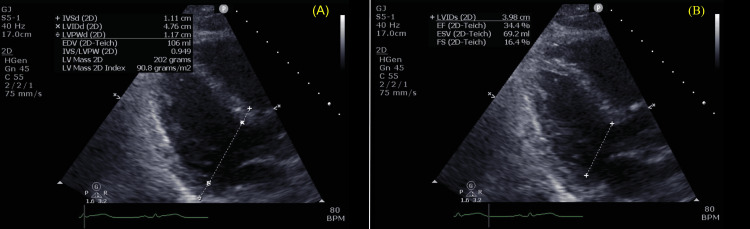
Transthoracic echocardiogram demonstrating systolic dysfunction (A) Modified four-chamber view at end-diastole. (B) Four-chamber view at end-systole. Teichholz-derived measurements demonstrate an impaired left ventricular ejection fraction (LVEF) of 34% and right ventricular dilation. These findings are consistent with systolic dysfunction and, in the clinical context of elevated troponin and ST-segment elevation, raise suspicion for acute coronary syndrome with a differential diagnosis of immune-related myocarditis. Abbreviations: IVSd, interventricular septum thickness end-diastole; LVIDd, left ventricular internal diameter end-diastole; LVPWd, left ventricular posterior wall thickness end-diastole; EDV, end-diastolic volume; LV, left ventricle; LVIDs, left ventricular internal diameter end-systole; EF, ejection fraction; ESV, end-systolic volume; FS, fractional shortening.
